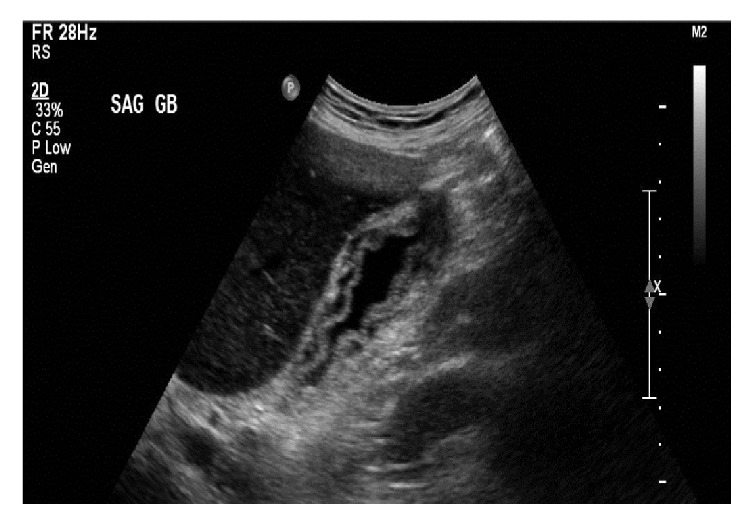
Grayscale longitudinal sonographic image of the right upper quadrant shows a decompressed gallbladder wall with diffuse wall thickening. The gallbladder wall has a striated appearance without increased vascularity.
